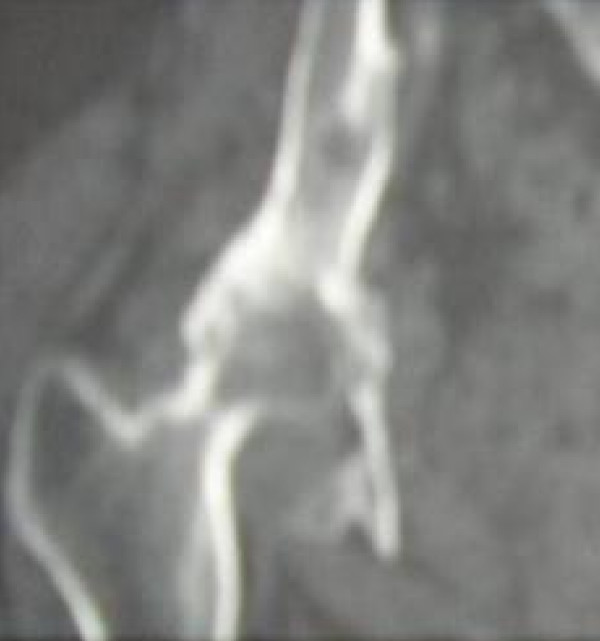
CT scan showing destruction of the hip with superior migration of the femoral head into the iliac bone affected by the hydatid disease.
